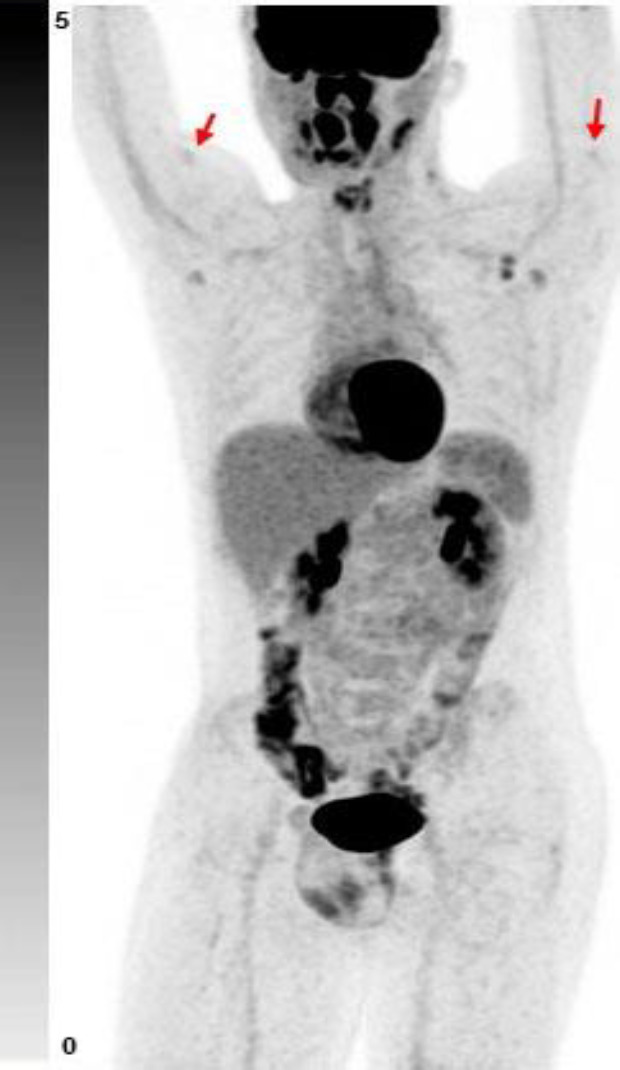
A 26-year-old gentleman with post-transplant lymphoproliferative disease presents for post chemotherapy response assessment. The patient had history of flu and Pfizer (2^nd^ dose) vaccination injected to the right and left arms 7 and 21 days before the PET scan respectively. Bilateral metabolically active axillary lymph nodes are seen. While the Pfizer vaccination was 14 days earlier than flu vaccination, the nodal reaction on the left side is more intense compared to the contralateral side. The left axillary lymph nodes measure up to 10mm in short axis diameter with SUV_max_ up to 3.8, and the right axillary lymph nodes measure up to 8mm with SUV_max_ up to 2.3. Bilateral mild deltoid uptake (SUV_max_: 1.3 right, 1.4 left) within the injection sites (red arrows) is also seen. Having more than one type of vaccination within the last month, the patient was excluded from the study
